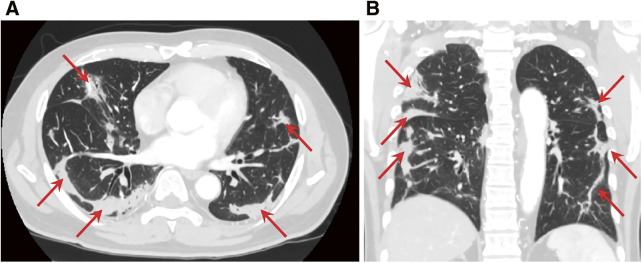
Fig. 2 Axial (A) and coronal (B) views of chest CT on first presentation. Red arrows show multiple areas of consolidation in peripheral regions of both lungs, which indicates post-COVID-19 pneumonia. COVID-19: coronavirus disease 2019
